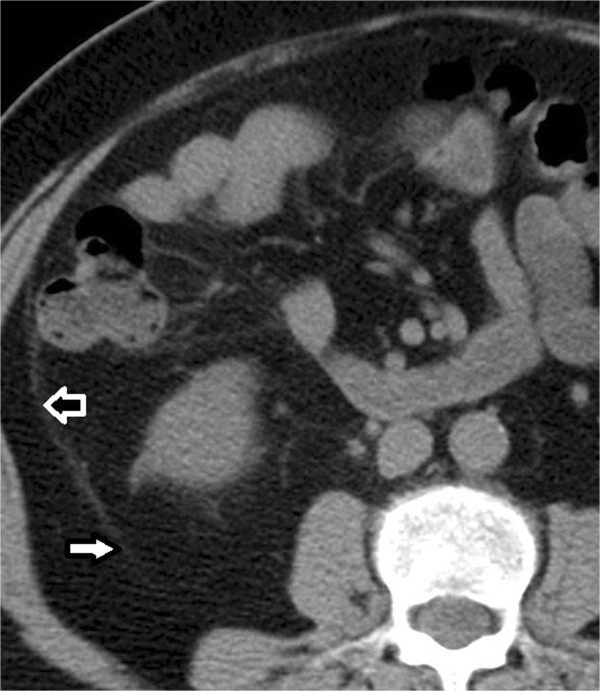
Transverse CT image of 59-year-old man with complicated appendicitis. Retromesenteric plane, retrorenal plane (white arrows), and lateral conal plane (black arrow) were involved.
